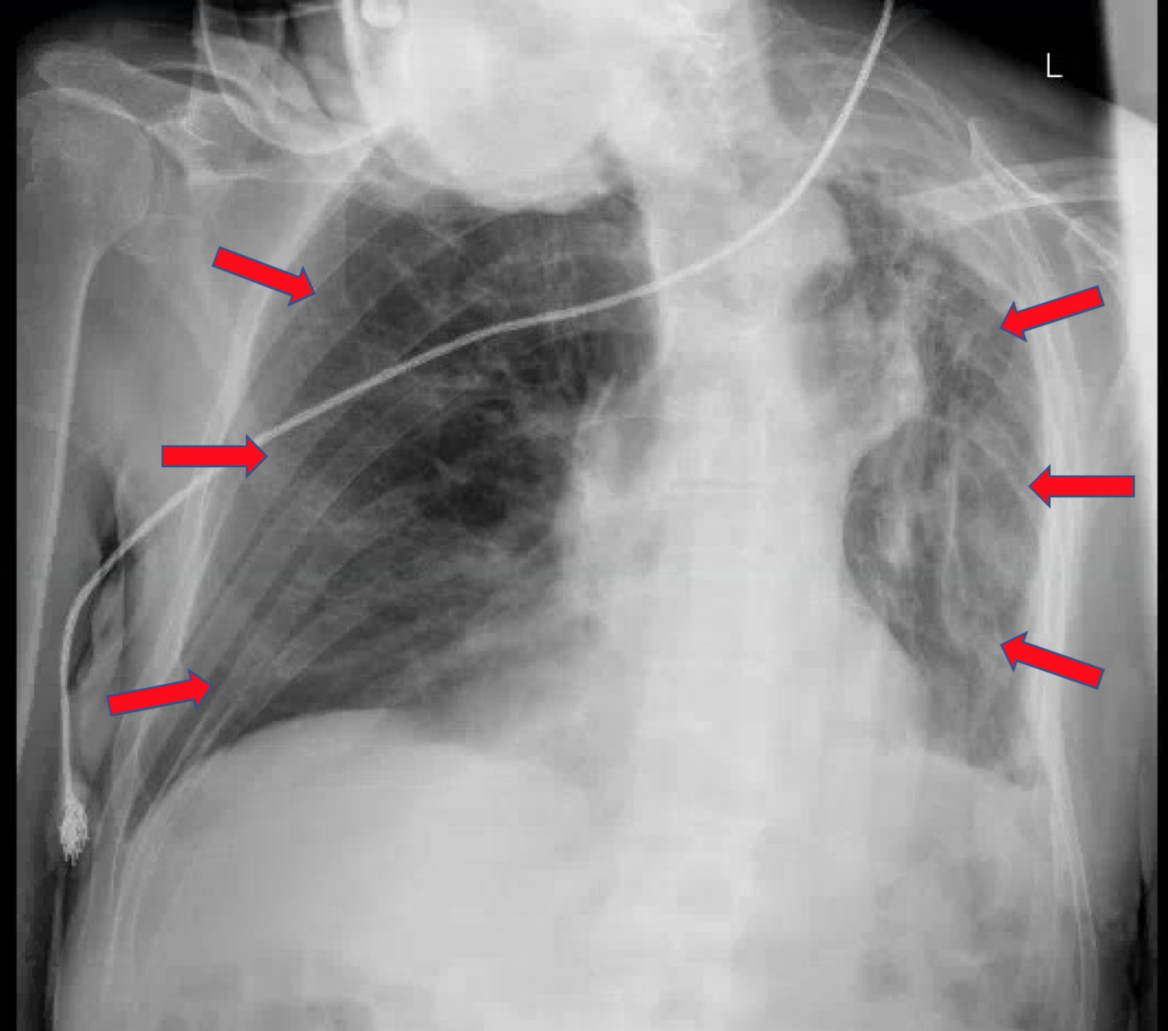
Chest x-ray on Day 9 of hospital course, after transfer from the intensive care unit (ICU) to the COVID-only medical floor, showing continued decrease of lung opacities bilaterally (arrows) with improved visualization and near resolution of right lower lobe consolidation (arrows)
